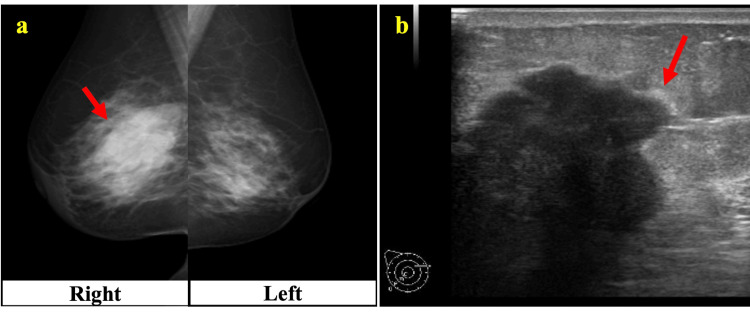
Imaging findings of breast cancer a: Mammography, the left side shows the right breast, and the right side shows the left breast. An irregularly shaped mass with spiculated margins is seen (arrow). b: Breast ultrasonography, an irregularly shaped mass is seen (arrow).
